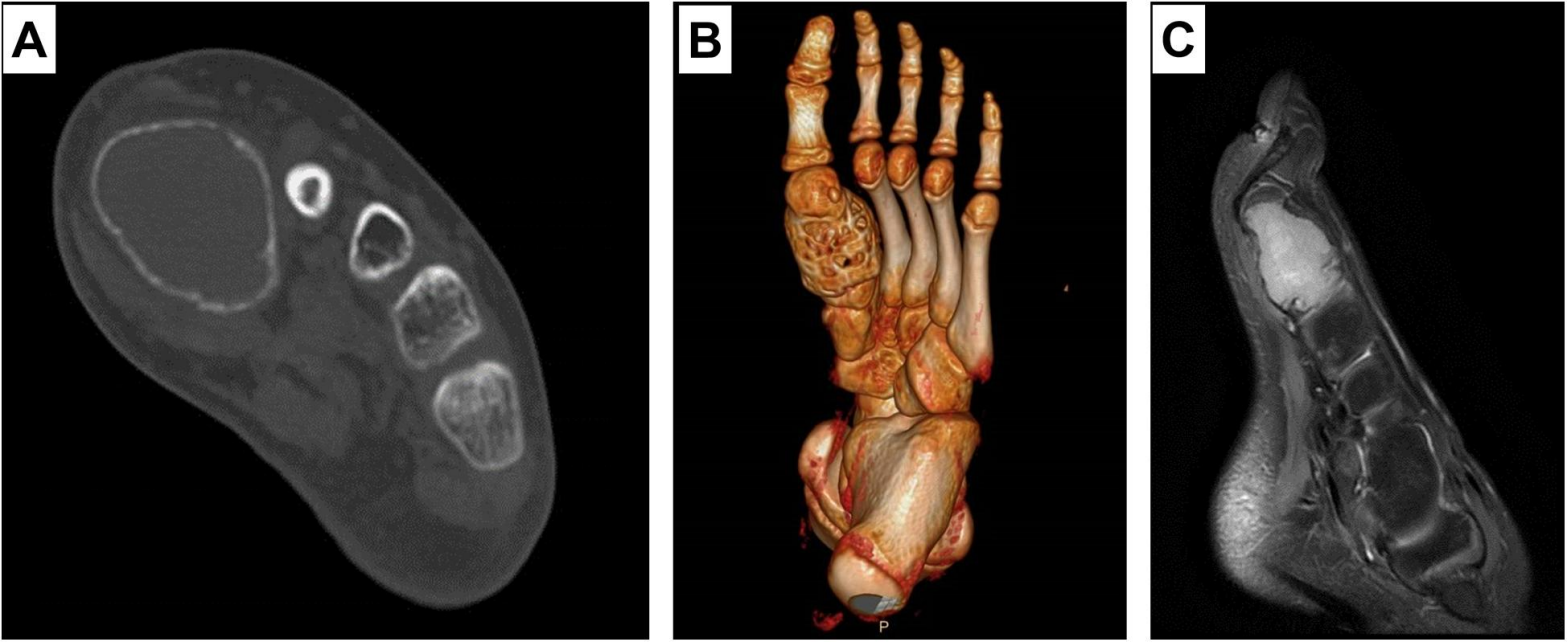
Preoperative CT (A,B) shows a space-occupying lesion in the first metatarsal bone, with expansile changes in the first metatarsal bone and thinning of the cortex; MRI (C) shows that the lesion invades the proximal articular surface of the first metatarsal bone, and a zone of bone edema can be seen at the distal end of the medial cuneiform.
